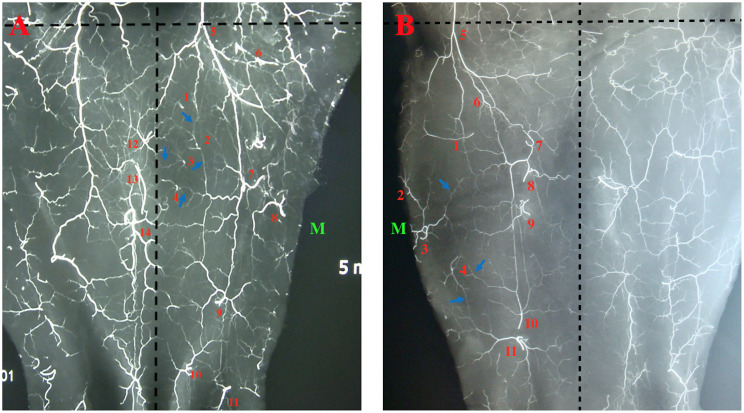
Radiograph showing arterial vasculature of leg integument [5, 6, 13]. (A) The integument was incised along intermalleolar line and anterior midline. Transverse and longitudinal dotted lines represent popliteal crease and posterior midline, respectively. (B) The integument was incised along intermalleolar line and posterior midline. Transverse and longitudinal dotted lines represent popliteal crease and anterior midline, respectively. Adjacent perforators of the medial sural artery (1, 2, 3, 4) are interconnected by choke vessels. The perforators of the medial sural artery (1, 2, 3, 4) form choke anastomoses anteriorly with the perforators of the posterior tibial artery (1, 8, 9, 10, 11), cross the posterior midline of the calf posteriorly, and laterally anastomose with the nutrient vessels of the sural nerve (14) and the musculocutaneous perforators of the lateral sural artery (12, 13) via choke vessels. The blue arrows indicate choke anastomoses. (5) Saphenous artery. (6) Medial inferior genicular artery.
